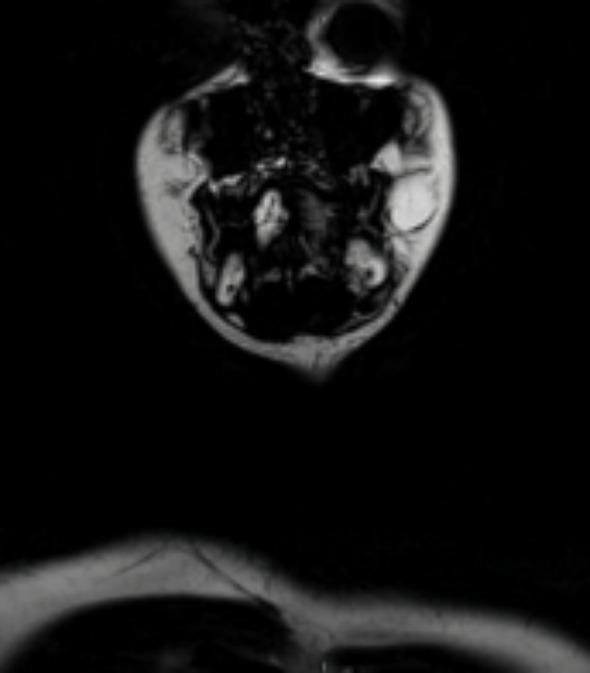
MRI Neck transverse (arrow pointing to tongue base tumour).
